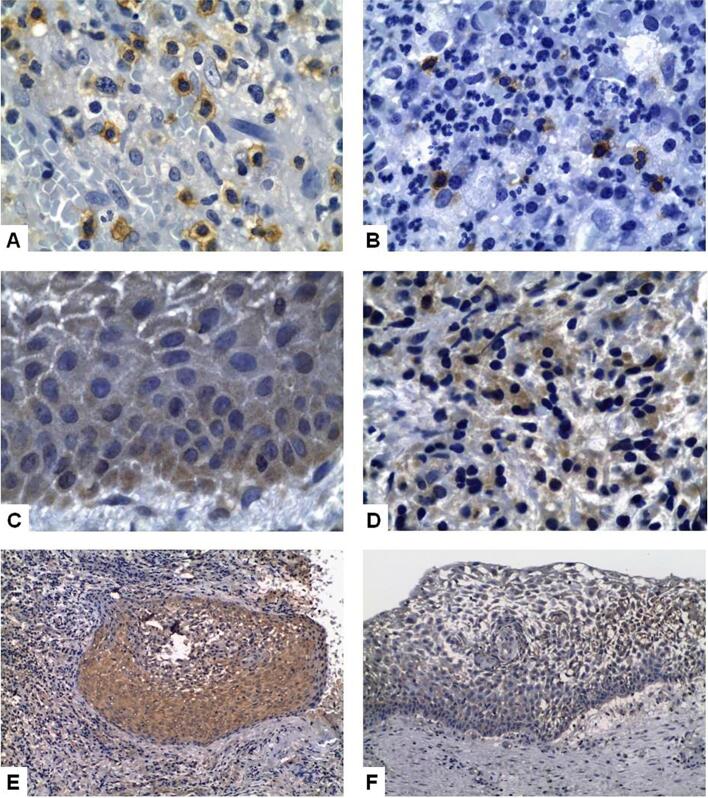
Presence of CD57+ cells in an inflammatory periradicular lesions with a chronic inflammatory infiltrate (A, Immunoperoxidase 1000x), and a mixed inflammatory infiltrate (B, Immunoperoxidase 1000x); Bcl-2 expression in the epithelium (C, Immunoperoxidase 1000x) and in the connective tissue showing chronic inflammation (D, Immunoperoxidase 1000x) in a periapical cyst; EGF expression in the epithelium of an initial periapical cyst (E, Immunoperoxidase 200x), and in the hyperplastic epithelium of an advanced periapical cyst (F, Immunoperoxidase 200x)
